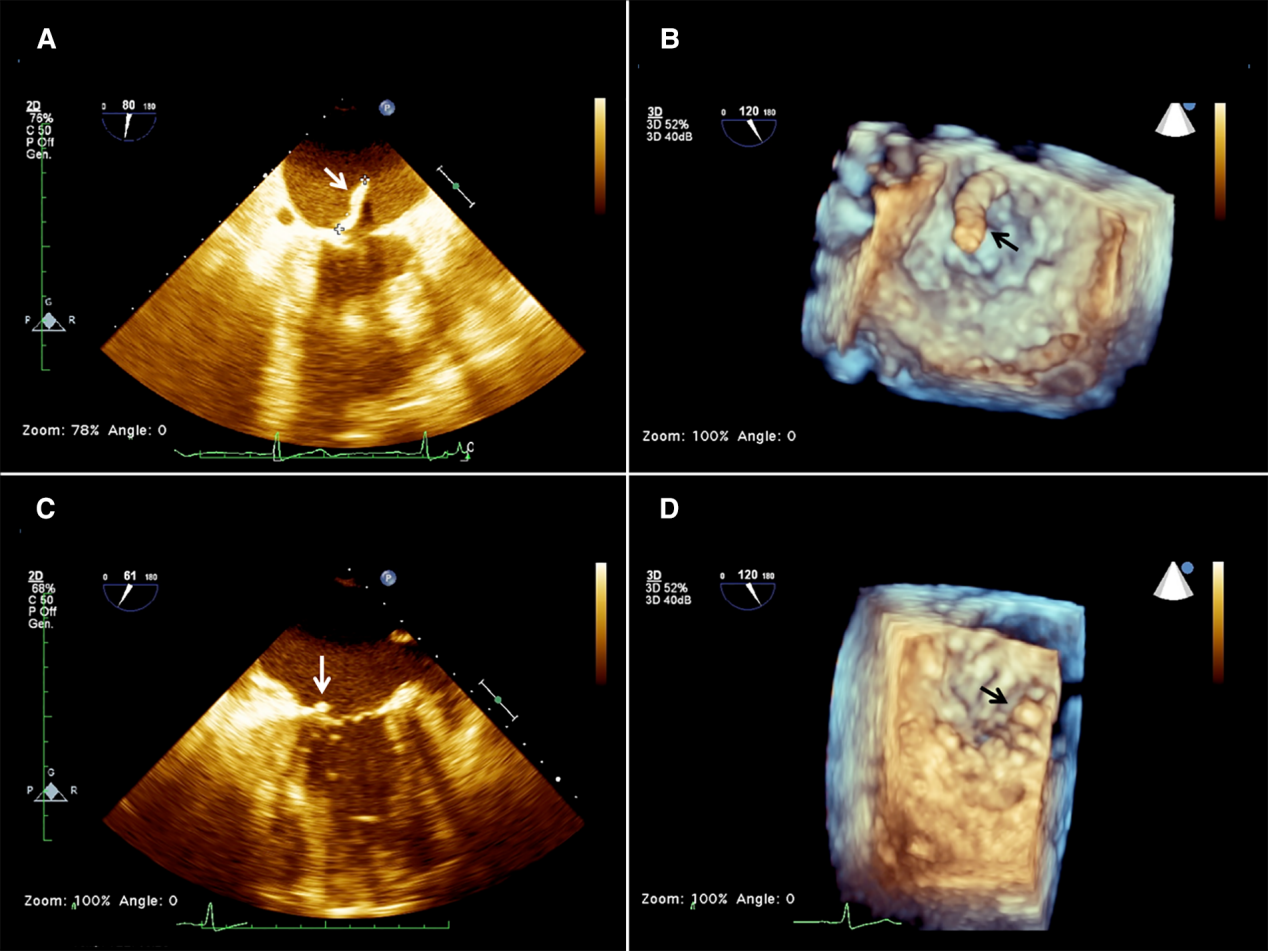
2d (A) and 3D (B) preoperative transesophageal echocardiography (TEE) showing the presence of a mass (arrow) attached to the base of the anterior mitral leaflet on the atrial aspect of the mitral valve, and 2D (C) and 3D (D) postoperative TEE showing a residual minimal stump (arrow).
